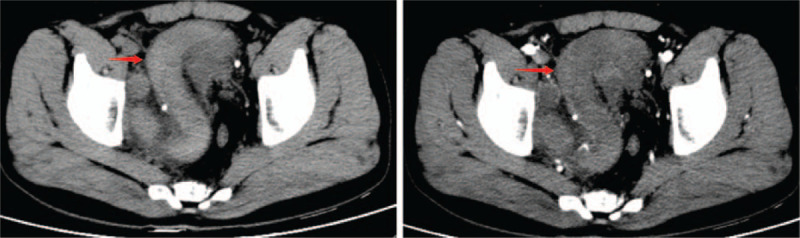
CT scan of the pelvis showing an “S” tube on the right side of the pelvis with a CT value of about 58 to 70 HU. The enhanced scan seemed to show a slight uneven enhancement, with slightly blurred fat spaces and a little exudation, and the prostate and seminal vesicle glands moved left under pressure.
